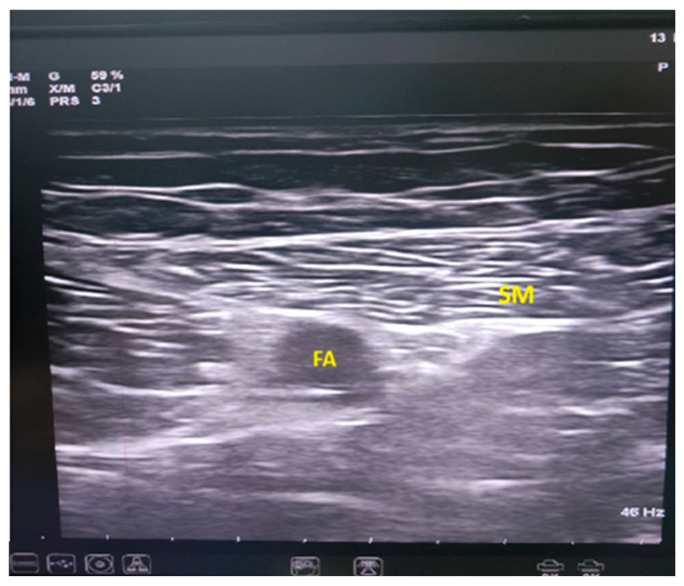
Ultrasound Image of the Proximal Adductor Canal Block (FA: Femoral artery, SM: Sartorius muscle).
